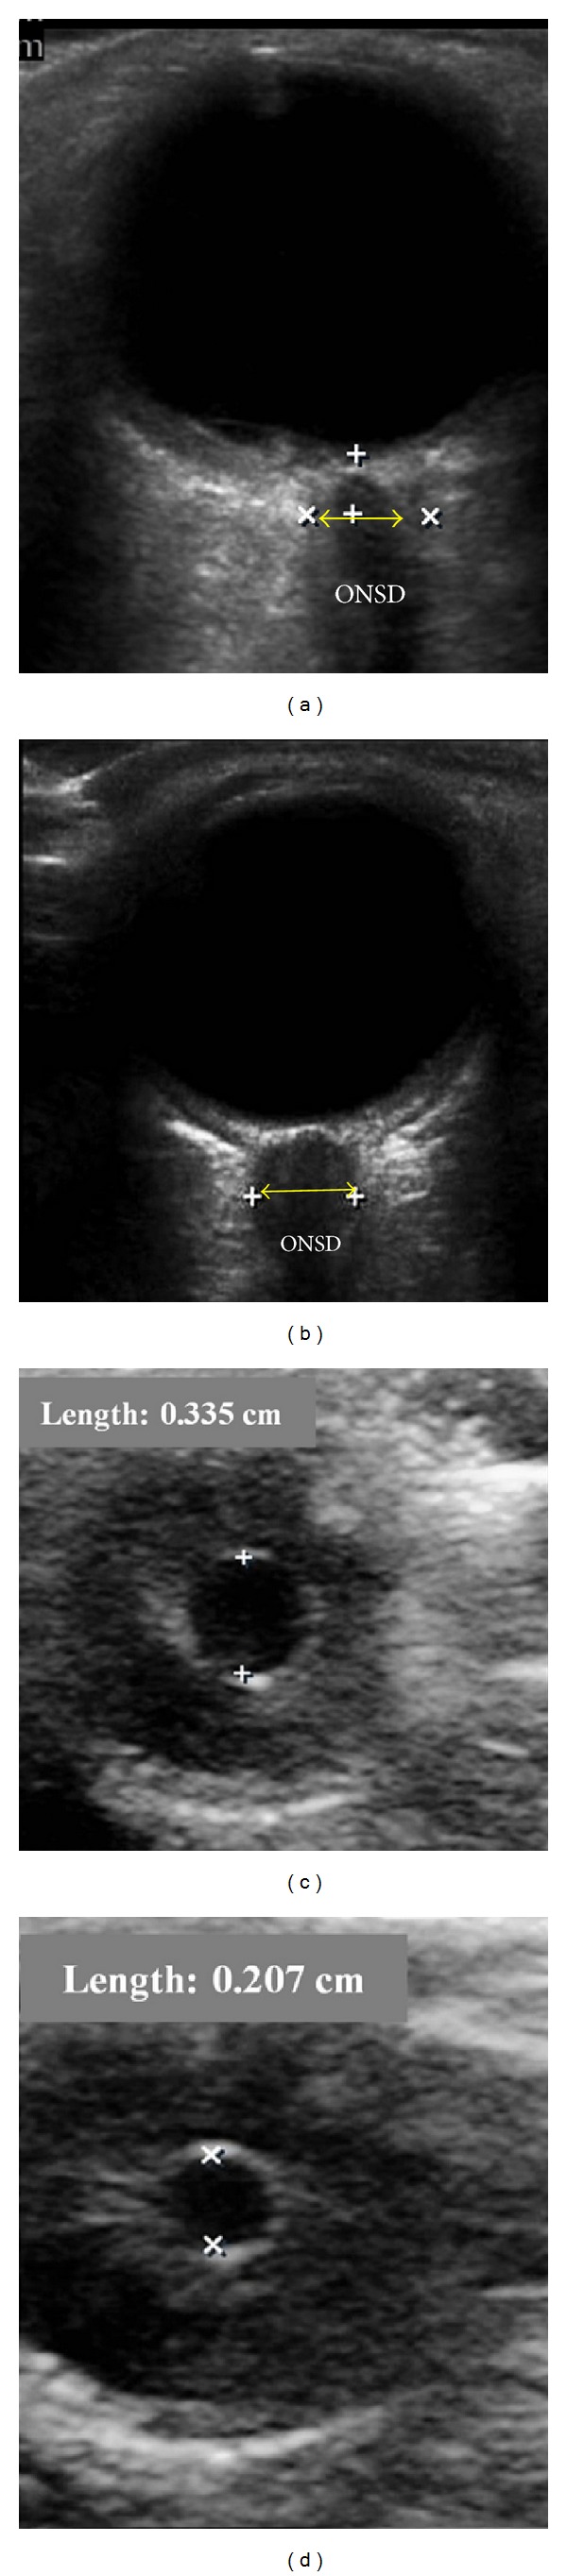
Optic nerve sheath: (a) normal diameter and (b) large diameter that represents increase intracranial pressure. ONSD: optic nerve sheath diameter. It is usually measured 3 mm behind the retina. Ultrasound pupillary light reflex: (c) diameter of the pupil before shining light to the contralateral eye and (d) the pupil constricted after shining the light.
